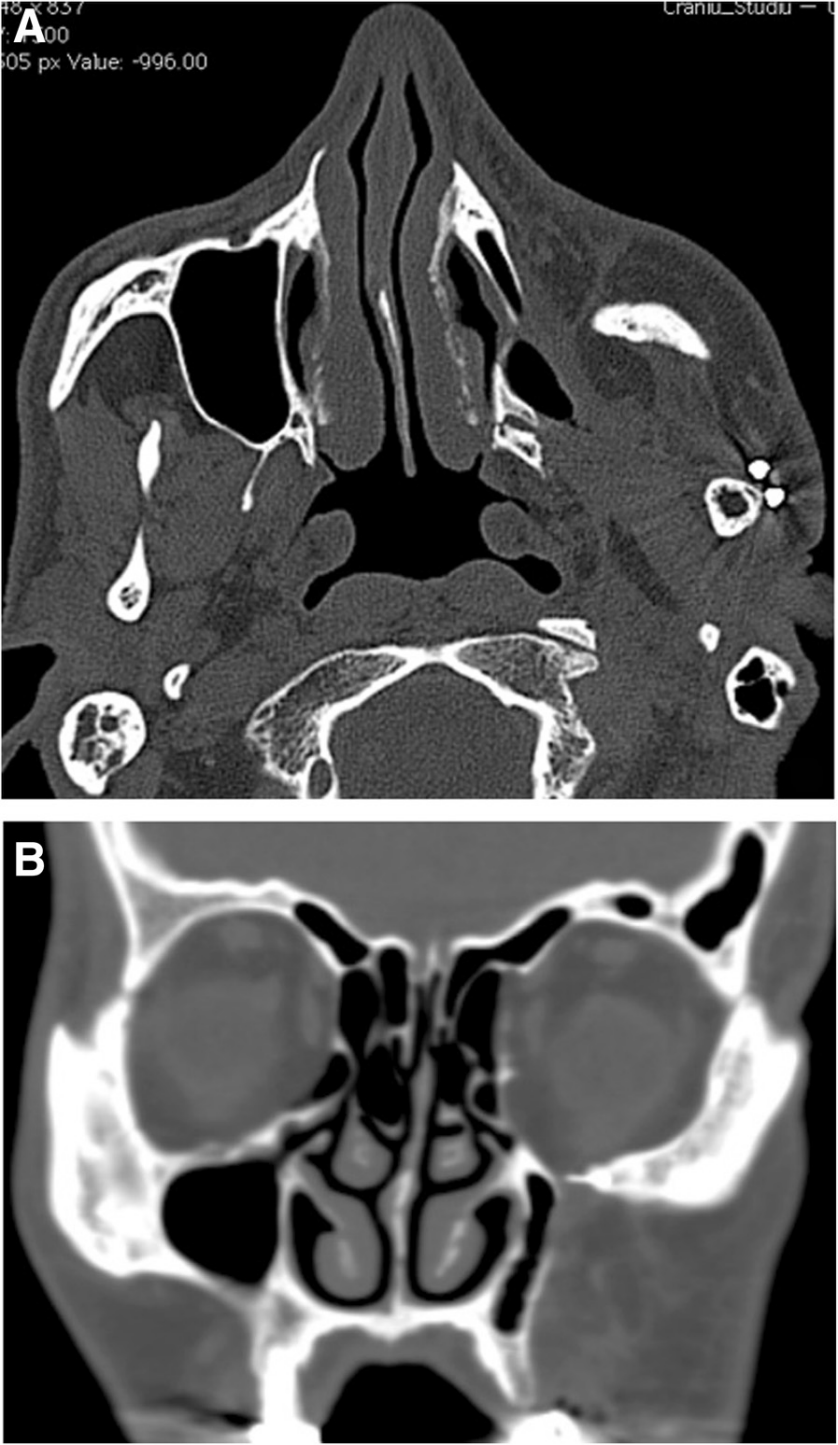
CT scan confirming the left zygomatic deficiency: (A) Axial, (B) Coronal.
